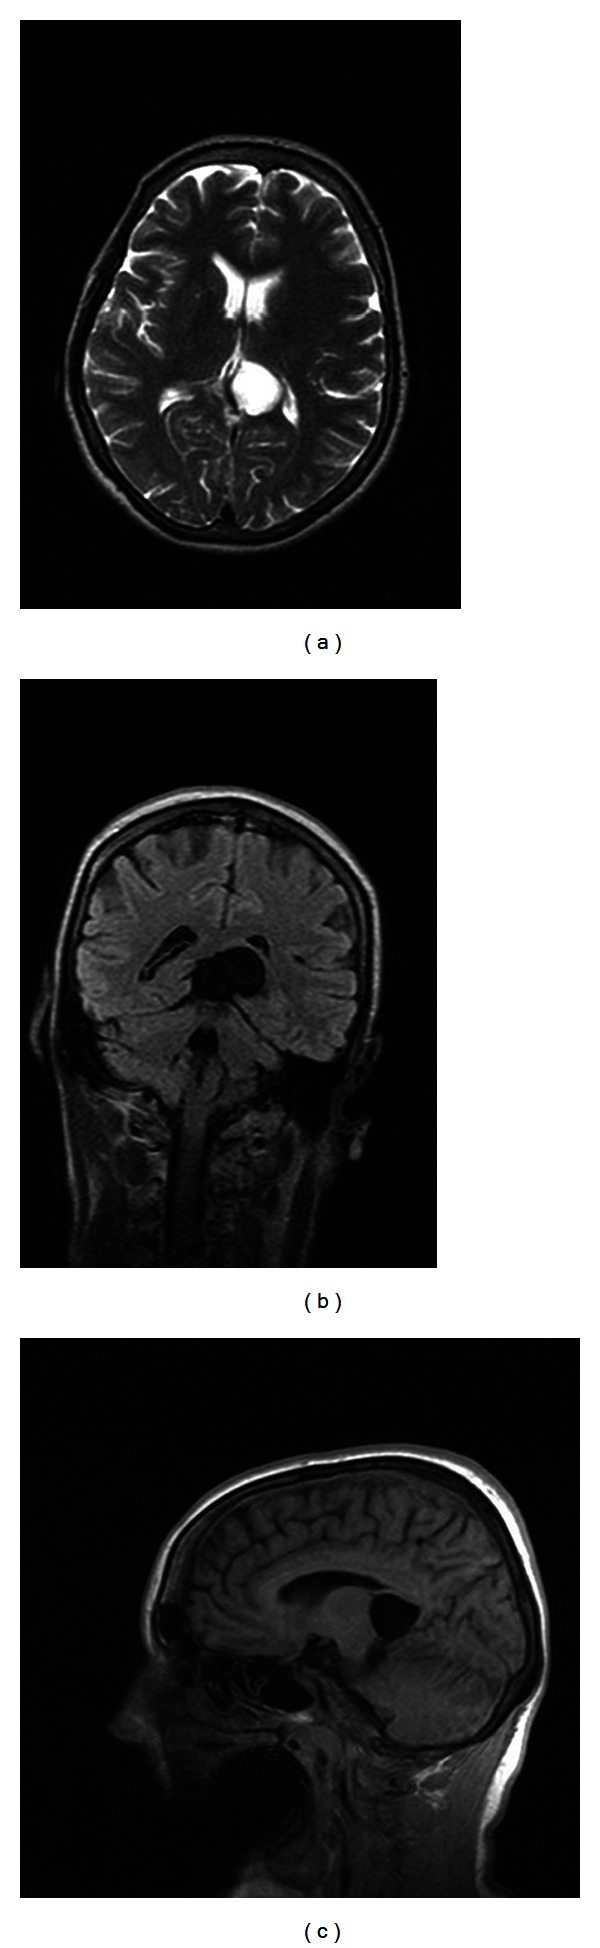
Cisterna ambiens arachnoidian cyst. (a) Round-ovalar, homogeneous, well-demarcated, hyperintense lesion with signal similar with CSF revealed by T2-weighted MRI image. (b) Round-ovalar, well-demarcated, hypointense cystic lesion with signal similar to CSF revealed by FLAIR MRI sequence. (c) Round-ovalar, homogeneous, well-demarcated, hypointense lesion with signal similar to CSF revealed by T1-weighted MRI image. Department of Neurology, Colentina Clinical Hospital brain imaging archive.
